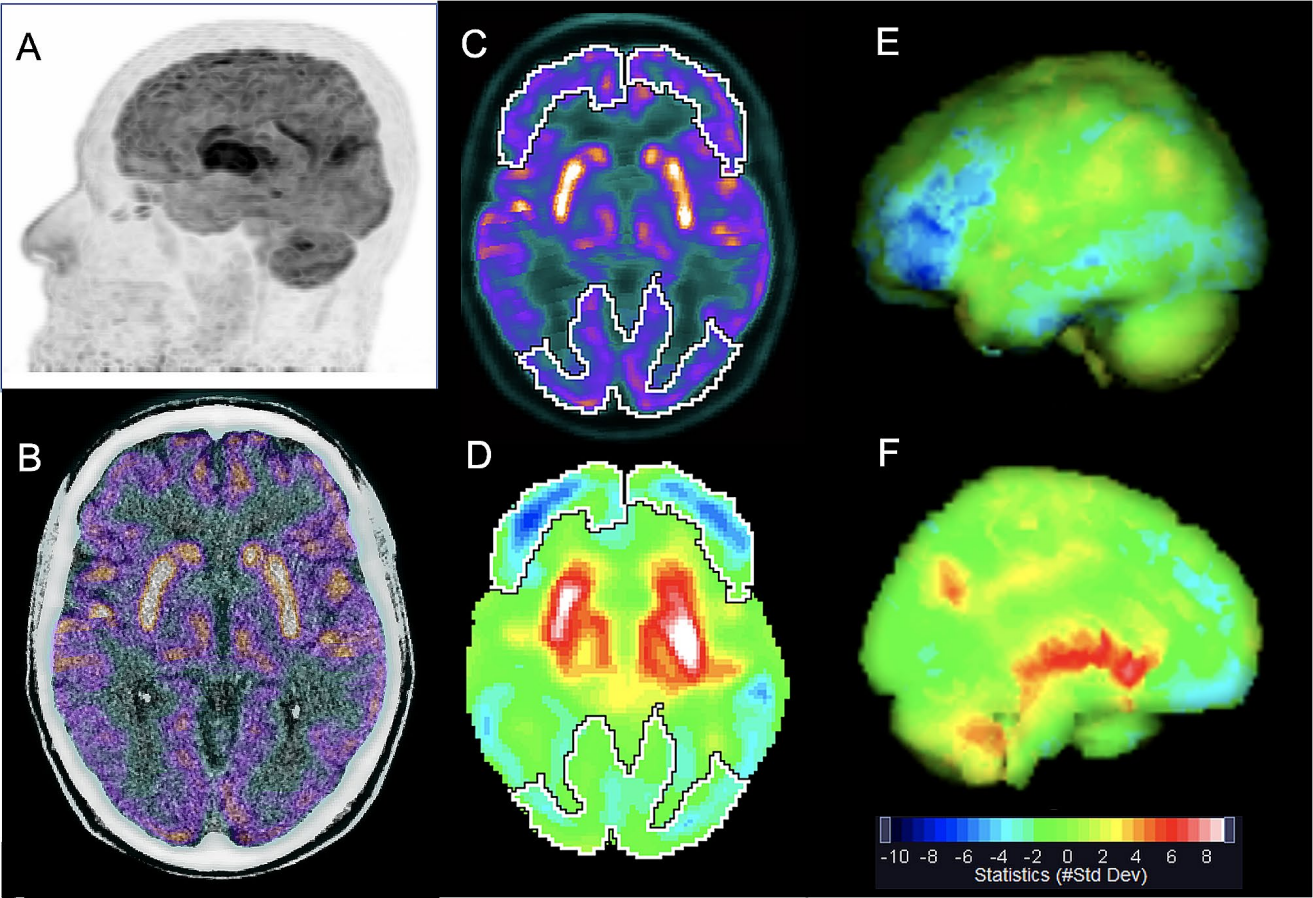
[^18^F]FDG PET/CT of a 69-year-old man on the 20th day of acute COVID-19, with respiratory failure, without neurological symptoms. Maximum intensity projection (MIP) (A) and axial PET/CT image fusion of the brain (B) show marked bilateral [^18^F]FDG uptake in lenticular nuclei. The axial plane of automatic delimitation of frontal and occipital lobes is shown in C and D. Quantitative data are displayed in a rainbow color scale as standard deviation (#StdDev) of the mean uptake of a normal database: in an axial plane in D, and as three-dimensional surface rendered displays of the left hemisphere of the brain in E (lateral view) and F (medial view). Reduced metabolism is seen in frontal lobes and temporo-occipital areas (respectively dark blue and light blue) in A and E. Also note the markedly increased brain metabolism in lenticular nuclei and thalami (red in D and F), suggesting a redistribution of brain metabolism from the neocortex to evolutionary ancient brain structures. During a 13-month follow up, no neurological symptoms or sequelae were detected. Quantification values (in #StdDev units) are: -4.6 / -2.6, for right / left frontal lobes; -3.3 / -2.4, for right / left temporal lobes; -3.0 / -3.9, for right / left occipital lobes; 11.5 / 12.5, for right / left lenticular nuclei; 3.7 / 4.8, for right / left thalami
